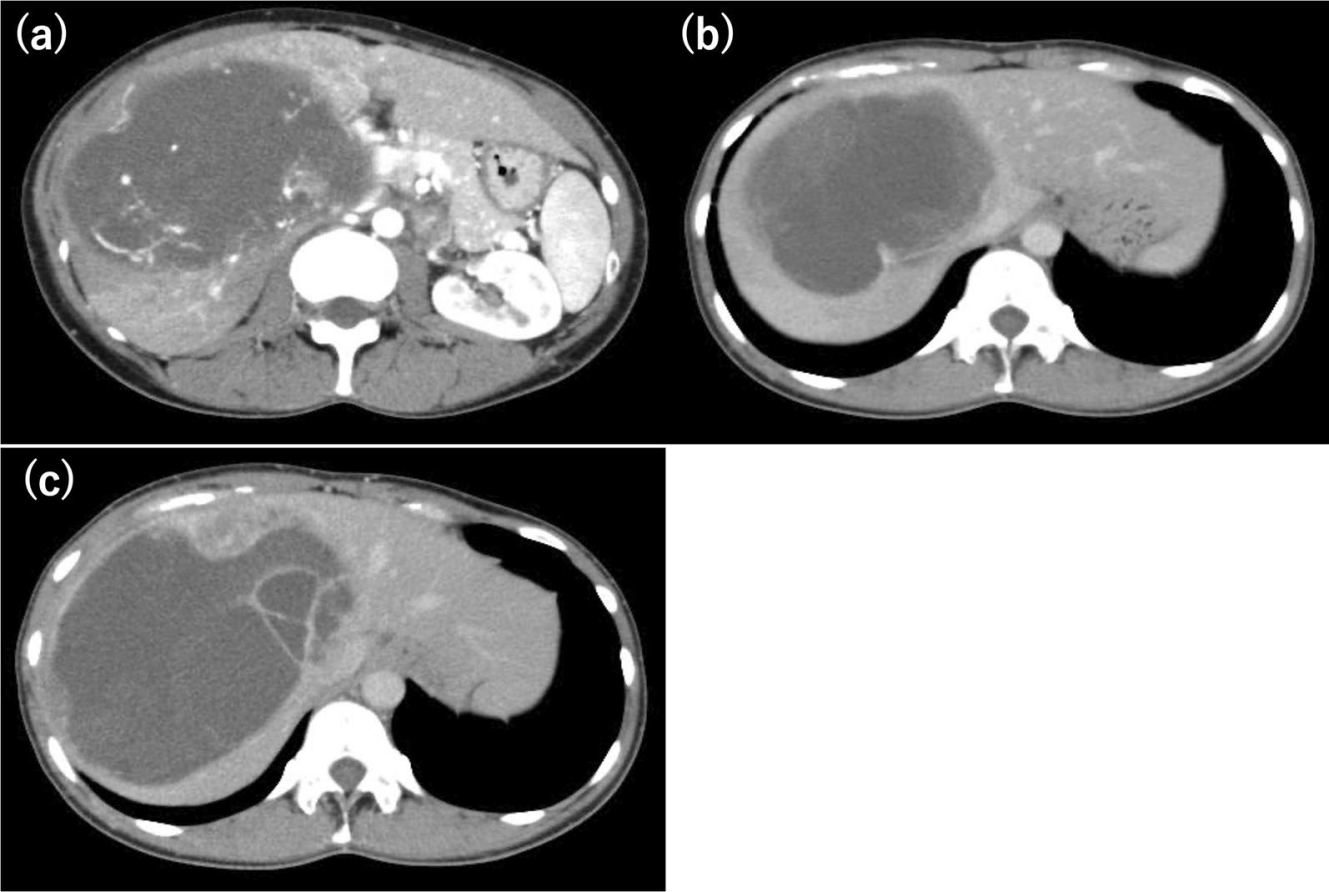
a and b Computed tomography showing a well-defined cyst. c At the time of exacerbation of abdominal pain, the tumor grows and its solid component is increasing
